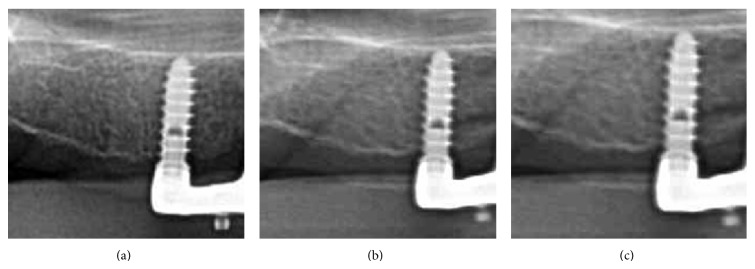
Periapical radiographs of an implant at the 1-year (a), 2-year (b), and 3-year (c) follow-up examination, respectively.
